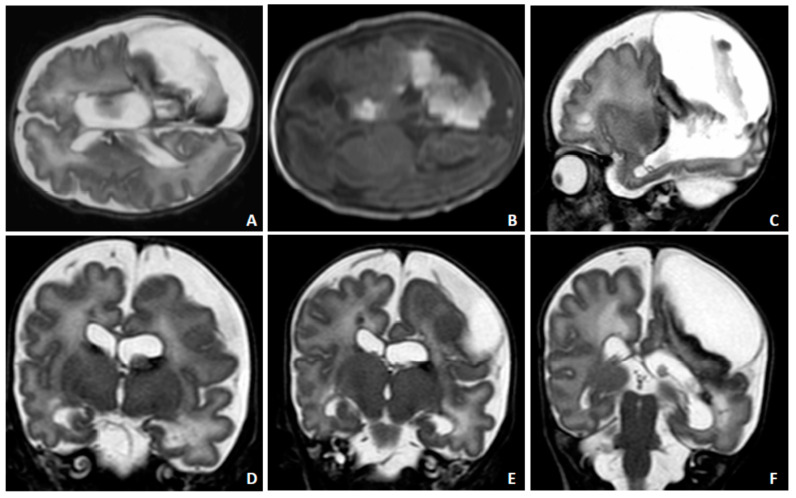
Neonatal MRI for Case 2 after 20 days of postnatal life. Chronic intraparenchymal and extraparenchymal hemorrhagic lesion with cystic appearance in the left cerebral hemisphere, ex vacuo ventricular enlargement (A–F) ((A,B): see paramagnetic component in T2 and T1 W images due to methemoglobin and hemosiderinic residual alterations). Persistent intraventricular septation with temporal horn cyst (C,E,F).
